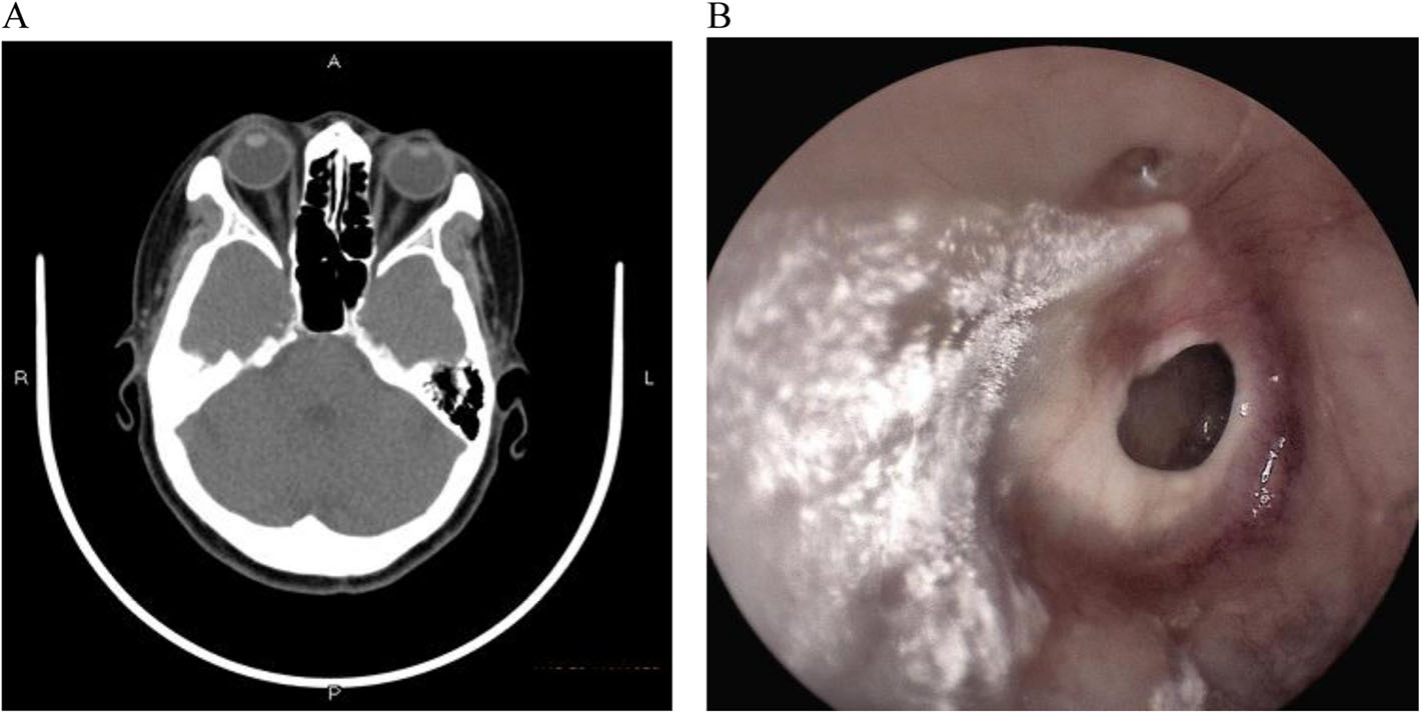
Computed tomography of mastoid process revealed chronic mastoiditis and tympanic membrane perforation of right side (a), and white aerial hyphae can be seen in the right external auditory canal, with large perforation in pars tensa of tympanic membrane in aural endoscopy (b)
